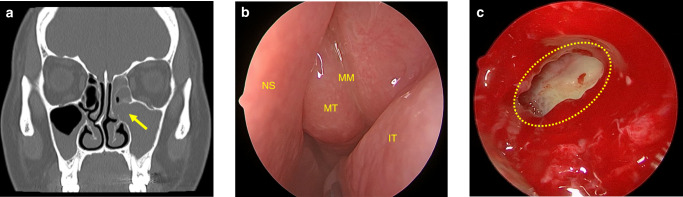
Maxillary sinus ostium obstruction caused sinusitis. a CT image shows maxillary sinus ostium obstruction (arrow) caused sinusitis. b Significant swelling of the mucosa of the middle turbinate and middle meatus can be observed. c The yellow circle points to the ostium and pus can be seen in the maxillary sinus in endoscopic operation. *IT* inferior turbinate, *MT* middle turbinate, *MM* middle meatus; *NS* nasal septum
